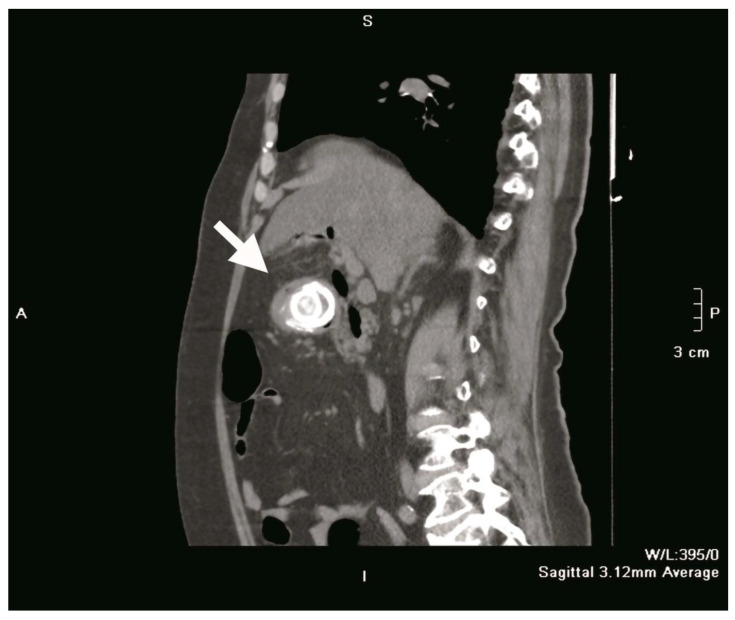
Sagittal section CT scan showing a gallstone (white arrow) impacted in the duodenal bulb.
